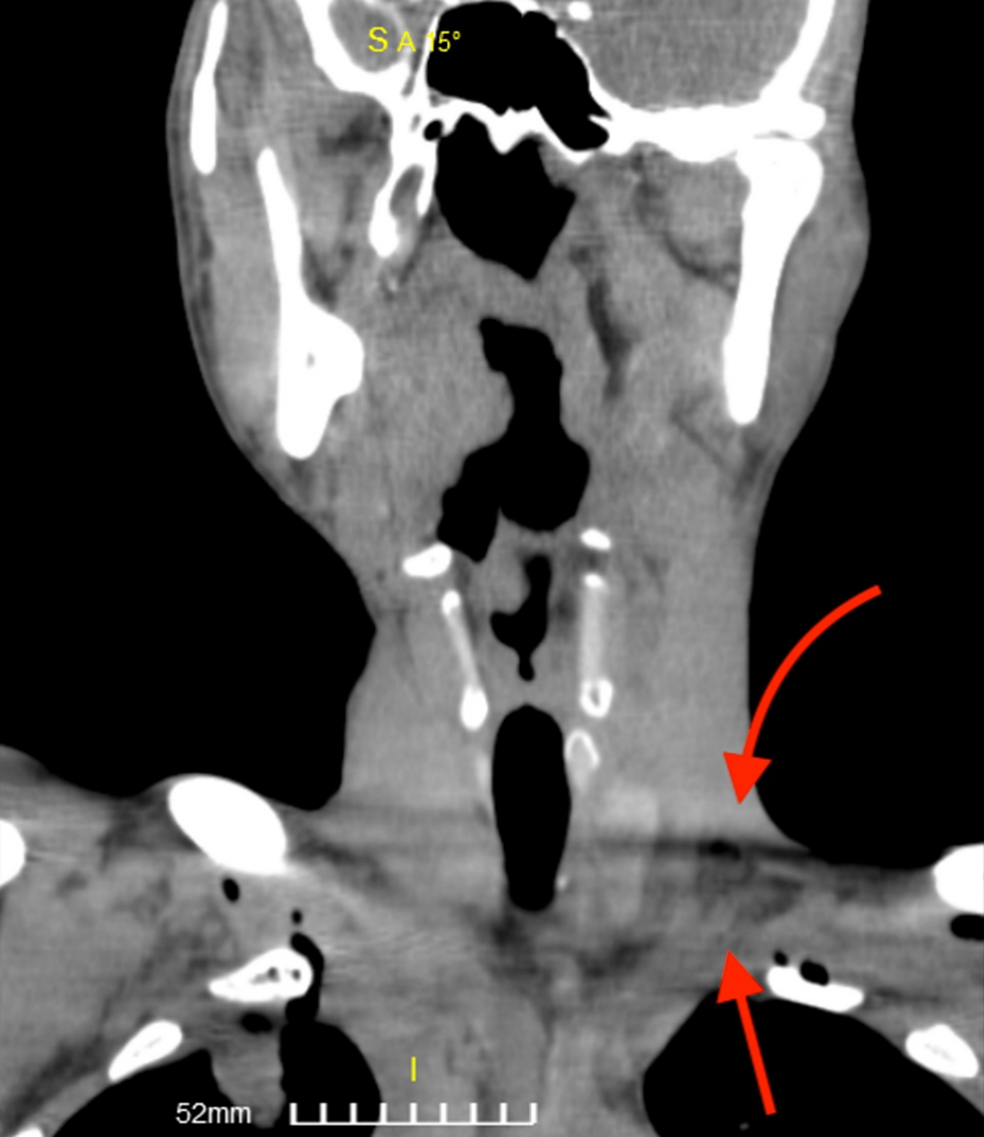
Neck CT scan, transverse view. CT: computed tomography
